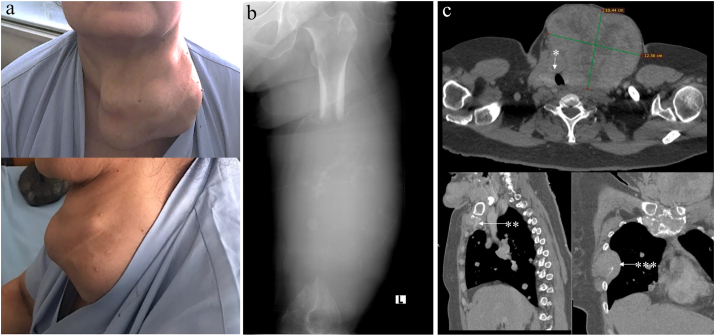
(a) A large multinodular neck mass was observed in a – 51 years old woman who presented in an oncology clinic with a gross deformity of the left side. (b) An expanded destructive lytic lesion in the left femur causing almost complete loss of the diaphysis was shown in X-ray. Some remaining bone fragments were observed within a large swelling of the surrounding soft tissues of the eroded bone. (c) Cervical contrast CT-scan showed a large thyroid mass of 12.5 cm in the diameter with tracheal deviation and 30% lumen narrowing (*). Thoracic CT-scan showed multiple lytic lesions in the manubrial sternum () and destructed 5th rib with expansive swelling (*).
